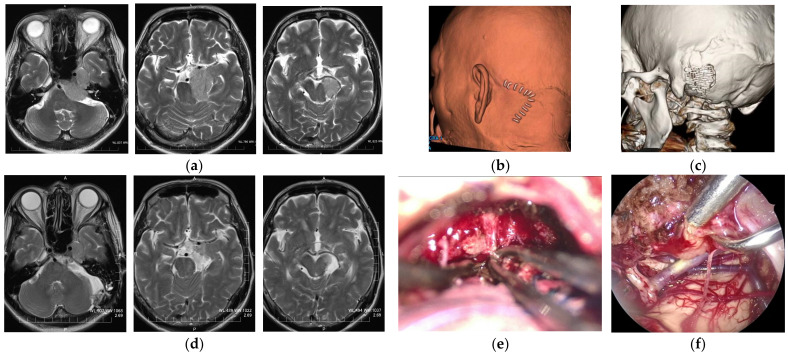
A 60-year-old male patient who complained of gait disturbance due to compression of the brain stem. (a) Axial T2-weighted images showing a right petro-clival meningioma. (b,c) Postoperative 3D CT images presenting a curved skin incision and retro-mastoid craniotomy fixed by a titanium plate. In this case, where the combined petrosal approach or staged surgery would have been selected in conventional microsurgery to achieve maximum resection, the tumor was partially removed by a lateral suboccipital approach using an exoscope (e), and the tumor in the ambient cistern that compressed the midbrain was removed by endoscopic observation (f). (d) Postoperative axial T2-weighted images showing the tumor removed, except the part in the cavernous sinus, while the compression of the brain stem was released.
